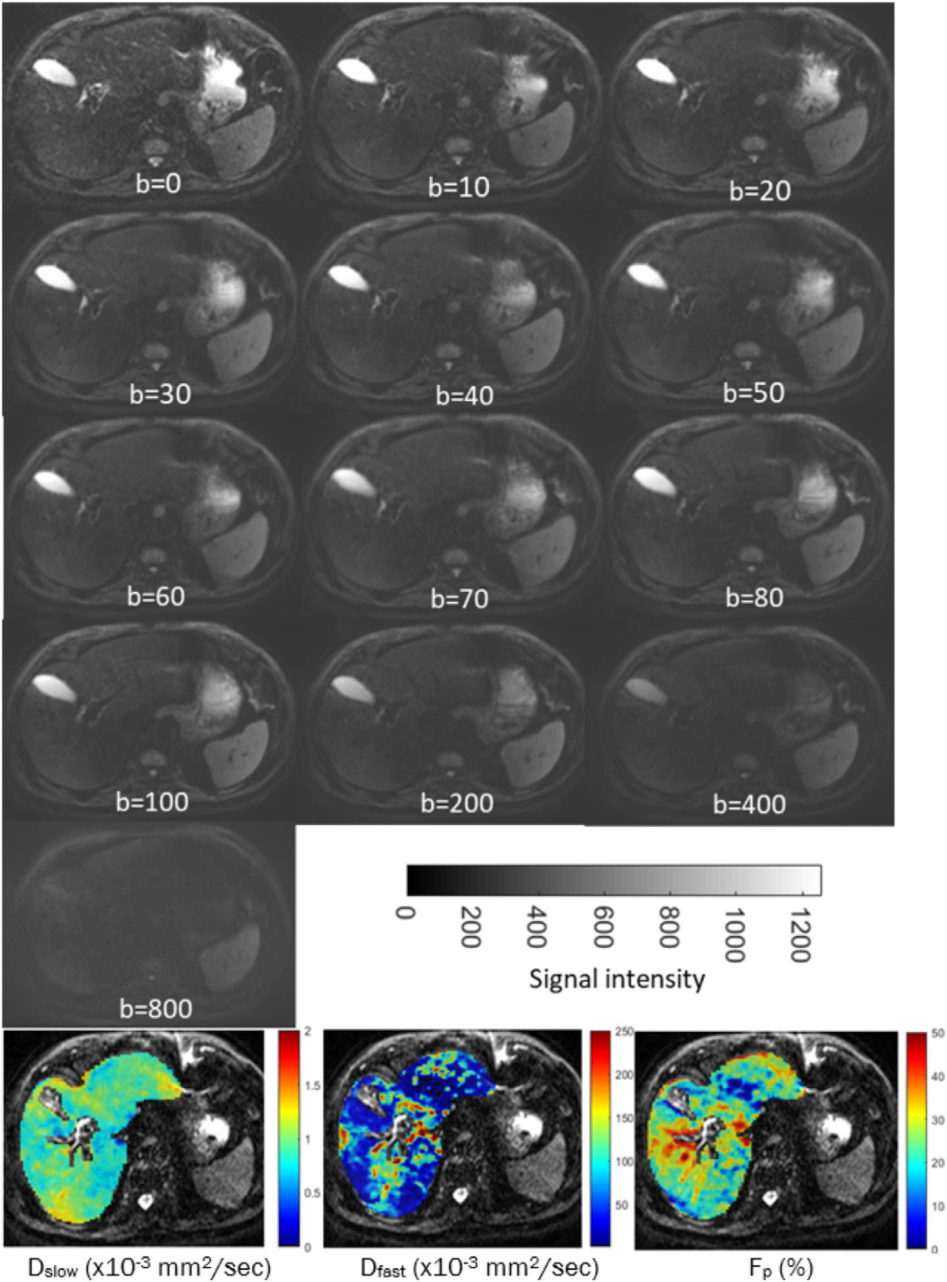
Example of 13‐b value IVIM‐DWI images with corresponding b‐value (s/mm^2^) and Bayesian‐based IVIM‐DWI derived parametric maps (*D_slow_
